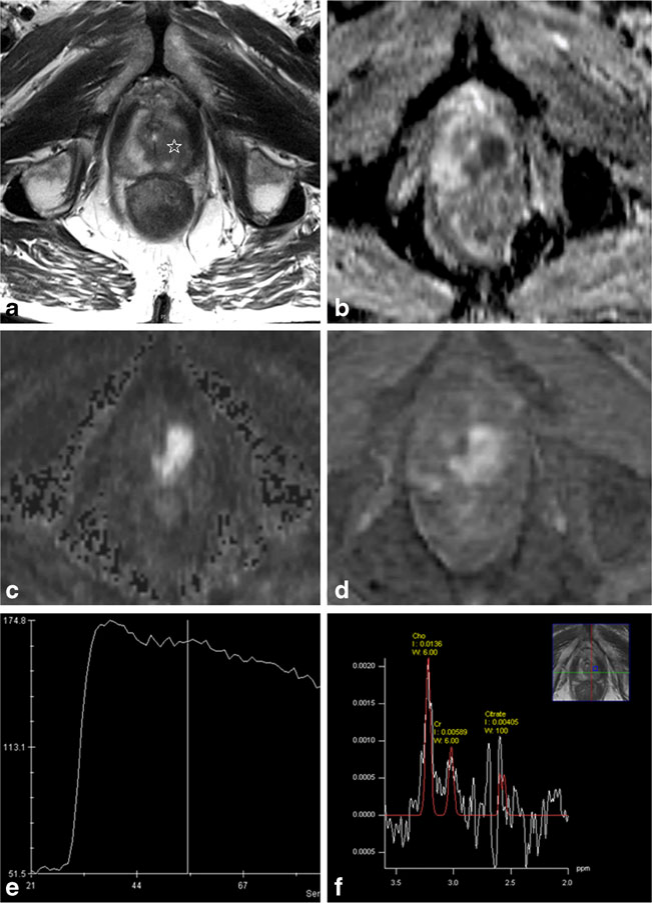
67-year-old man with persistently elevating PSA up to 6 μg/l. On this mpMRI a very suspicious lesion is detected in the apex of the prostate at the left side (white star). Morphologically it is demonstrated as an area of low SI with irregular margins on T2-WI (a), low ADC value (b) with high SI on the high-b-value image (c) of the DWI, strong contrast enhancement (d) with early and high peak enhancement with wash-out on the DCE curve (e). On MRSI the citrate peaks are reduced and the choline peaks elevated. The overall assessment score of this exam was PI-RADS 5. A targeted TRUS-biopsy with knowledge of the mpMRI findings (i.e., a MR-guided biopsy with cognitive fusion) confirmed a Gleason 4 + 5 PC in the apex at the left side
